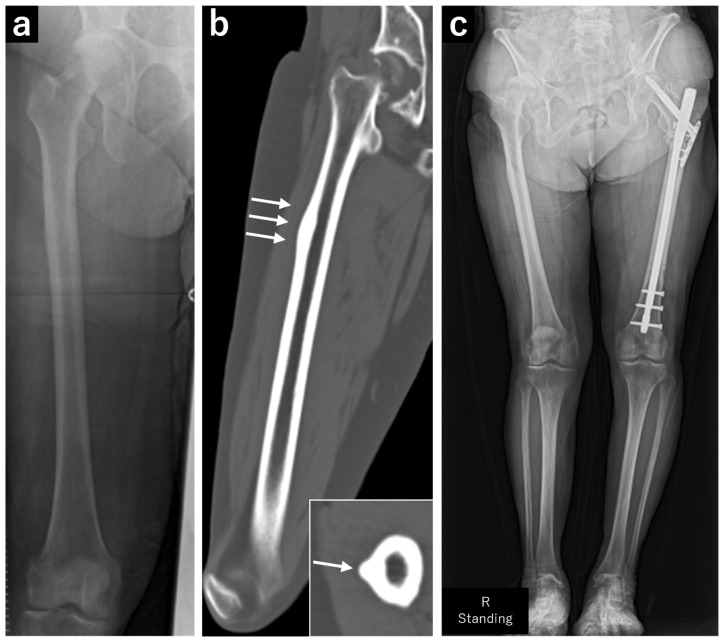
Plain radiograph and computed tomography (CT) images of the right femur. (a) Plain radiograph showing localized cortical hypertrophy at the subtrochanteric region. (b) Plain CT image showing no obvious radiolucent lines. The white arrows indicate localized periosteal thickening in the coronal and axial planes, respectively. (c) A full-length plain radiograph of the lower limbs showing no significant femoral bowing deformity.
